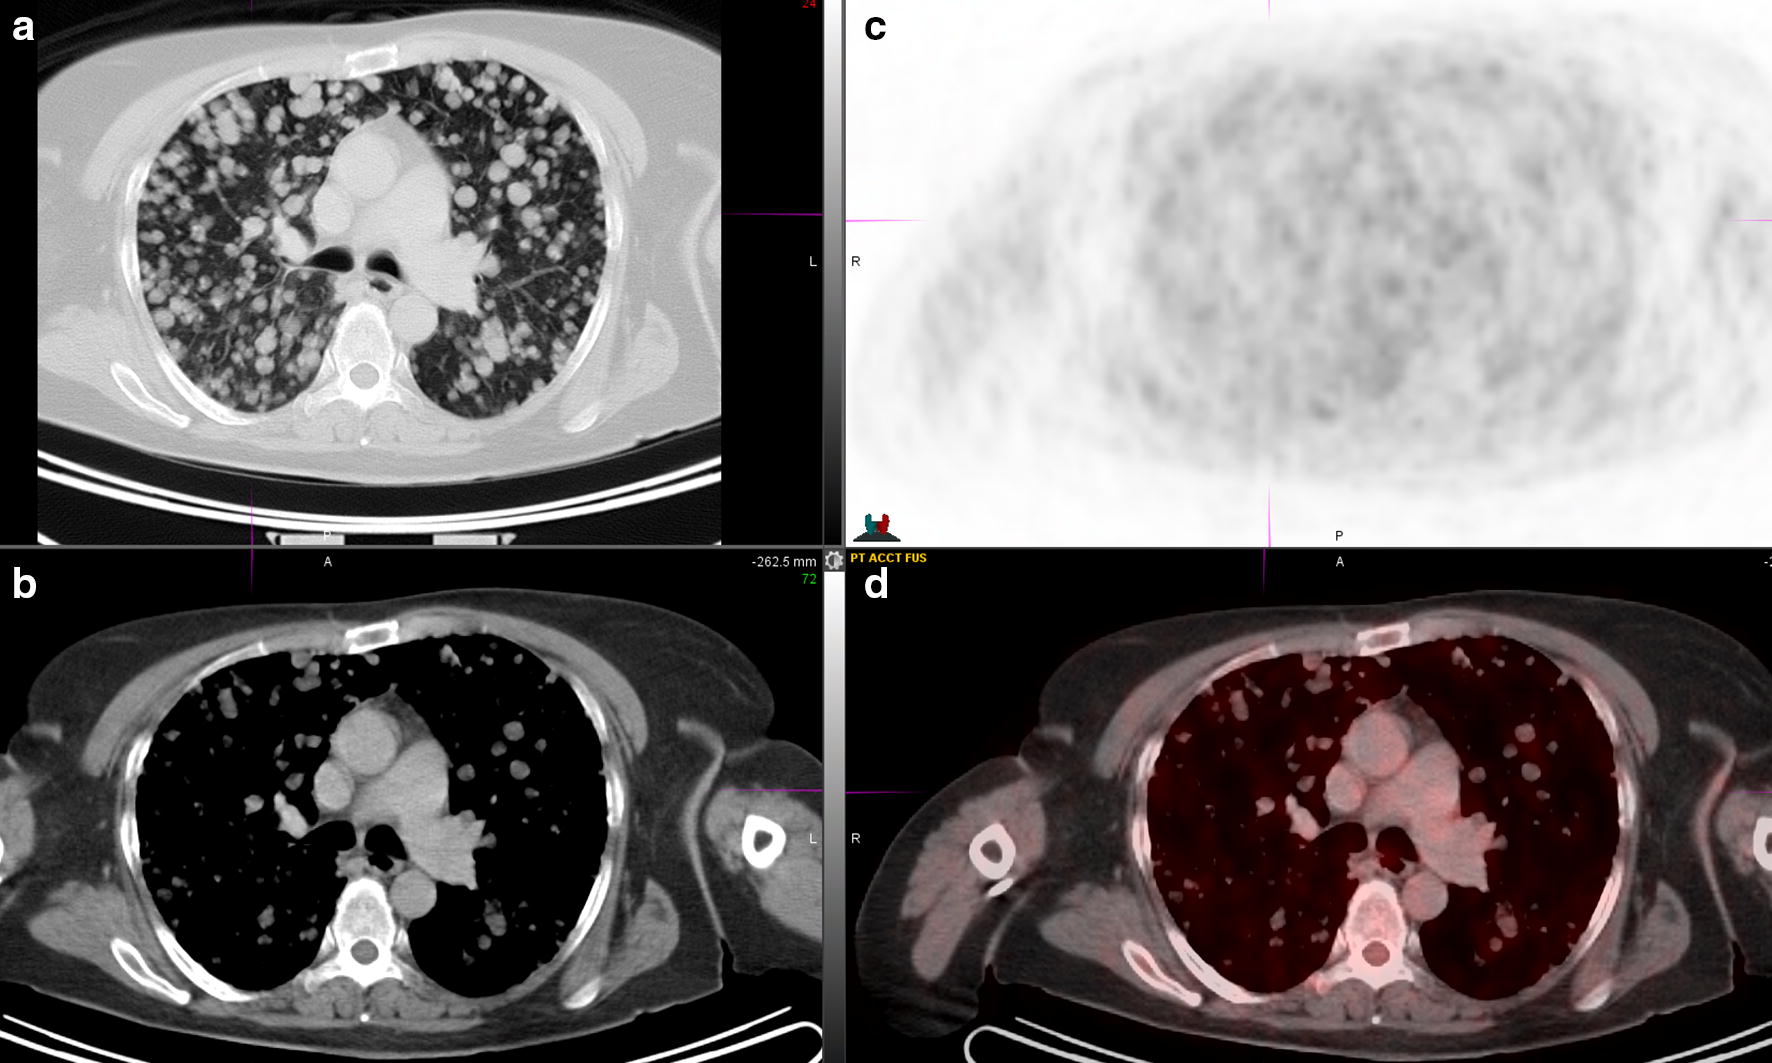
PET/CT at the time pazopanib was started. a CT lung window; b CT mediastinal window; c PET; d fusion CT and PET
